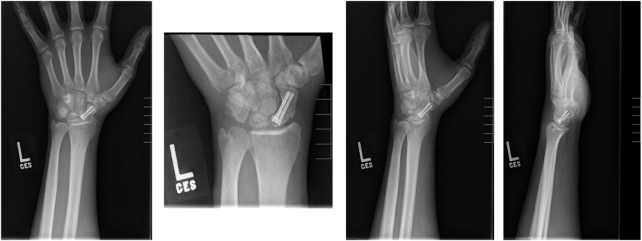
(Case 1, postoperative month 6): Four-view radiographs of the left wrist demonstrating the interval removal of K-wires, two retained scaphoid screws, and maintained carpal alignment without hardware complications.
